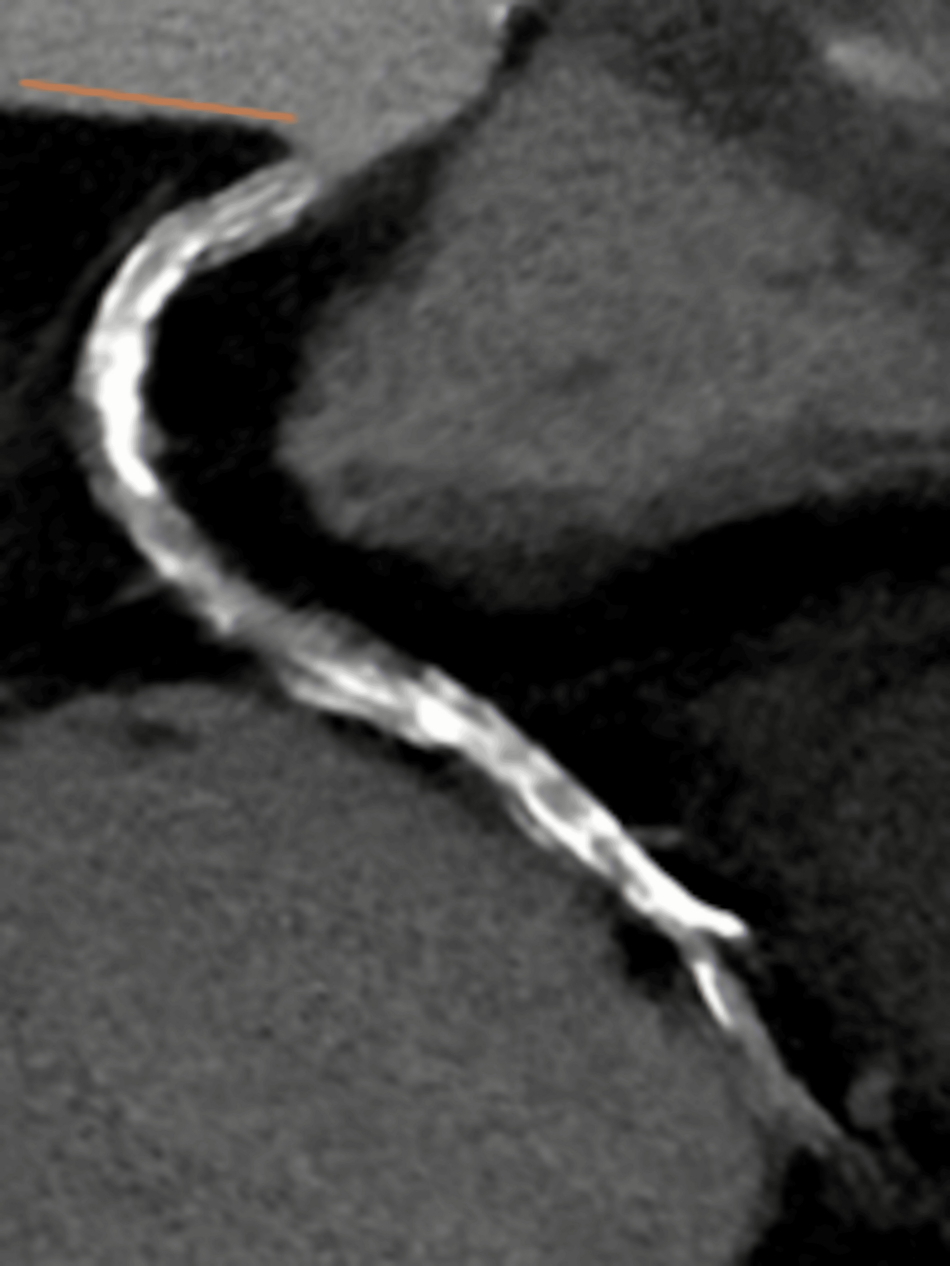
Axial contrast-enhanced computed tomography obtained 10 months after PCI. Axial contrast-enhanced CT obtained 10 months after percutaneous coronary intervention (PCI) shows no residual contrast within the sinus of Valsalva and no evidence of aortic dissection (orange line).
